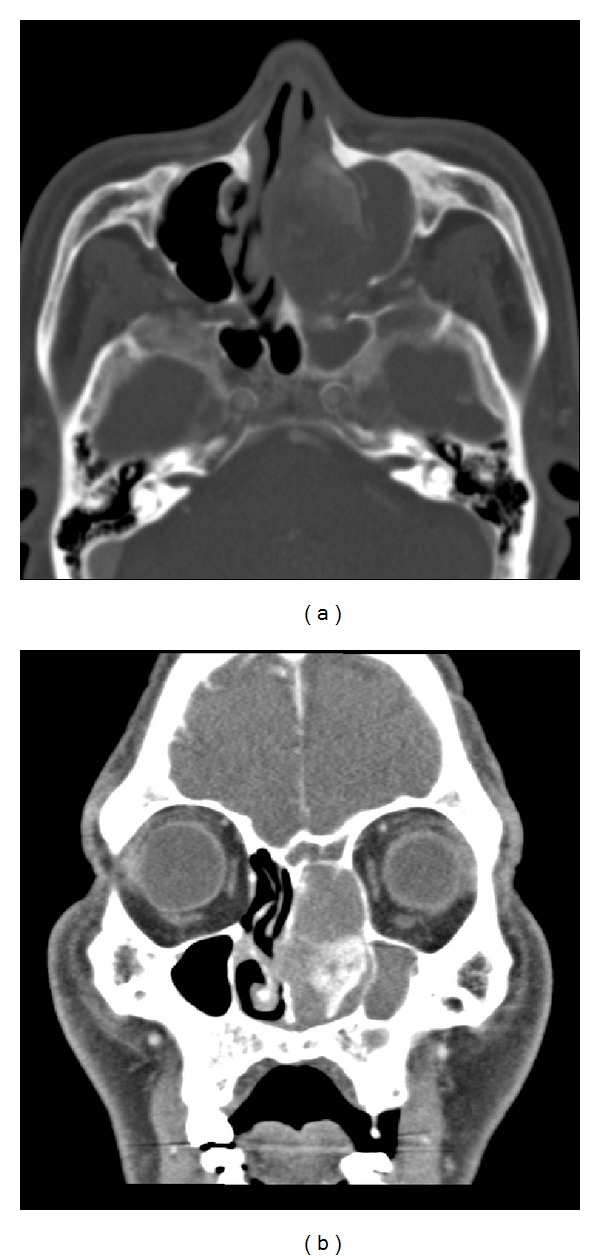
Preoperative computed tomography images showing a mass in the left middle turbinate as well as total opacification in all the sinuses: (a) axial view of the bone window; (b) coronal view of the mediastinum window.
